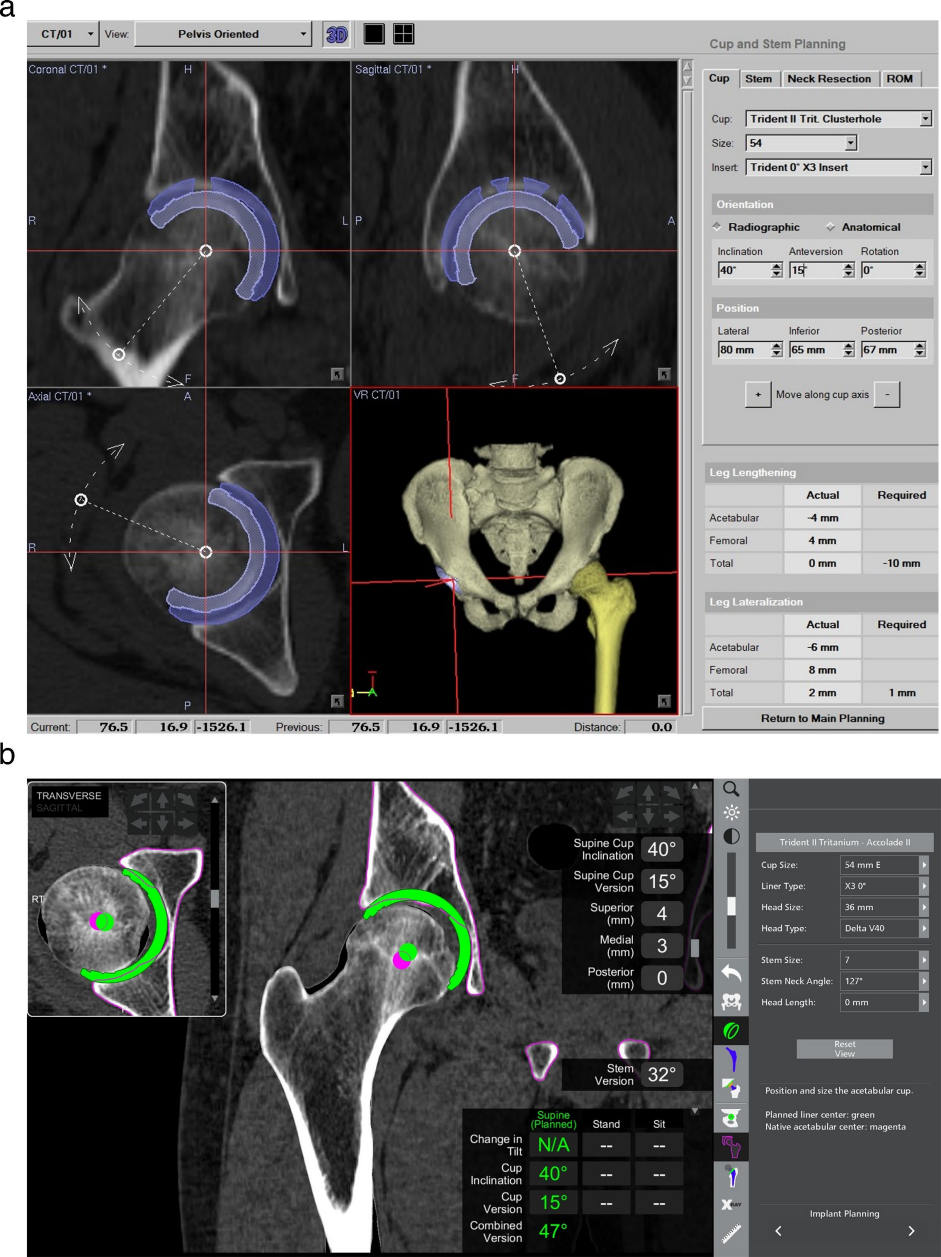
Preoperative planning was performed with a) a CT-based hip navigation system (Stryker CT-Hip System v. 1.1; Stryker, Germany), and then b) the data were accurately transferred to the Mako Total Hip application (Stryker, USA).
